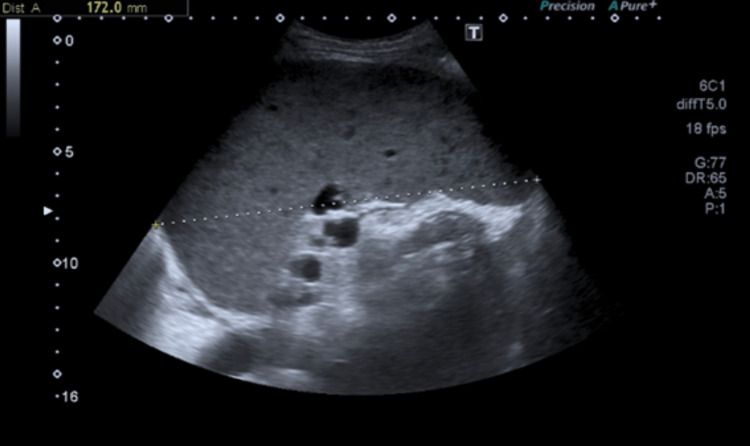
Abdominal ultrasound showing splenomegaly (splenic length of 17.2cm) with nodular images Cm: Centimeter
